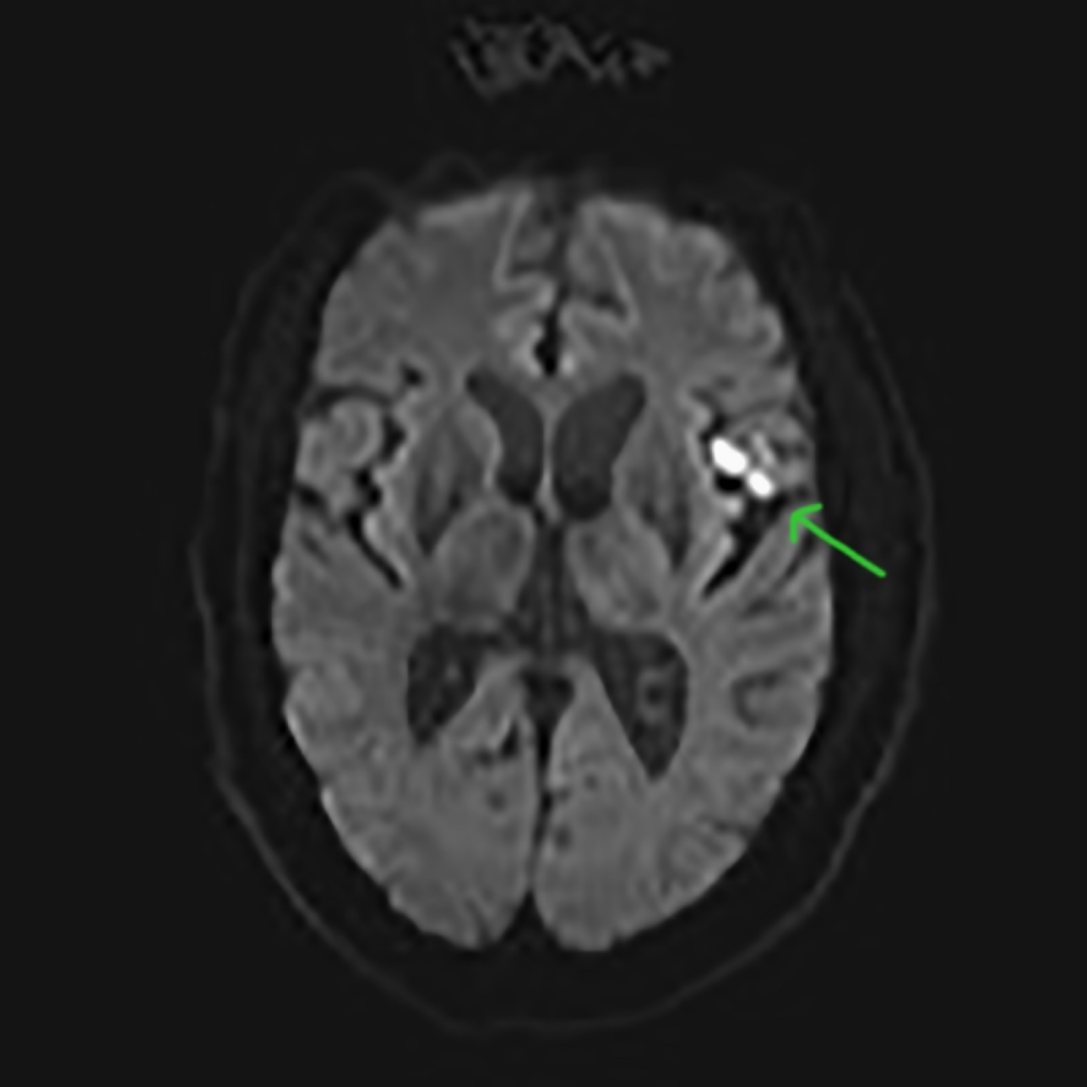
Magnetic Resonance Imaging (MRI) of the Brain Without Contrast MRI scan of the brain shows two small foci of increased signal intensity within the subcortical white matter of the left frontal lobe that represents subacute infarcts
